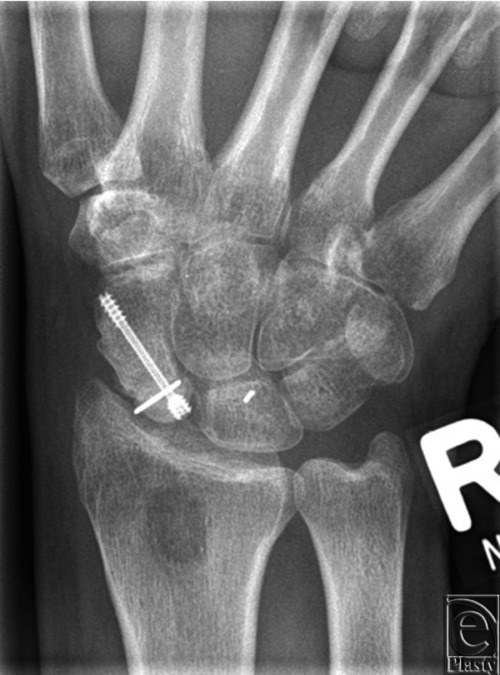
Posteroanterior radiograph showing the reconstructed and consolidated scaphoid after interposition of a dorsal vascularized bone graft from the distal radius. The graft has been additionally fixed by a 0.8-mm K-wire.
